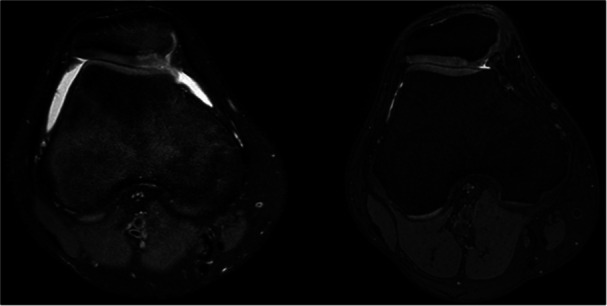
On the left, an axial pre‐perative magnetic resonance imaging shows a patellar cartilage lesion. On the right, the 5‐year postoperative magnetic resonace imaging after chitosan‐based scaffold implantation showed an improvement of most Magnetic resonance Observation of CArtilage Repair Tissue 2.0 variables evaluated.
